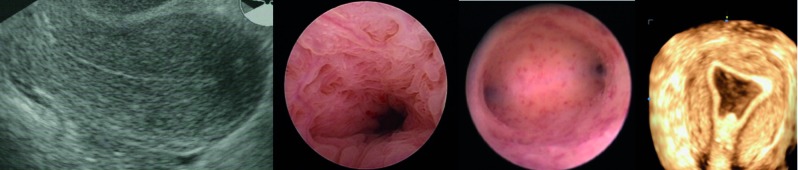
The one stop diagnosic exam includes transvaginal ultrasound (2 or 3 D) followed by hysteroscopy and contrast sonography of the uterine cavity.
